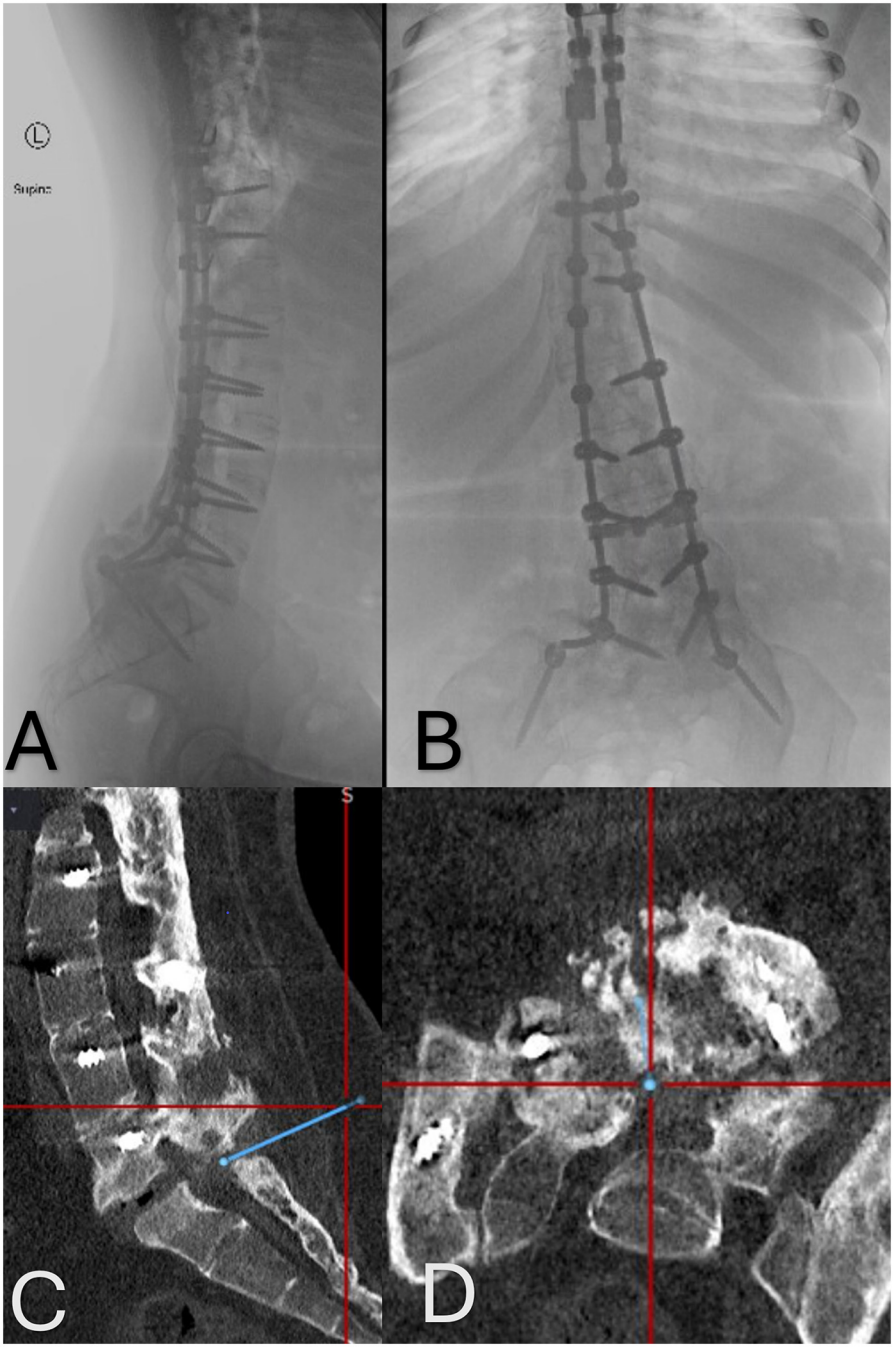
(A,B) Anteroposterior and lateral x-rays of the thoracolumbar spine demonstrating the extensive instrumentation utilized to stabilize the patient's spine. (C,D) Sagittal and axial CT images showing the significant bony fusion mass and the small area of pseudoarthrosis at the L5–S1 level. The blue line indicated the planned trajectory used for the placement of the Touhy needle into the intrathecal space.
